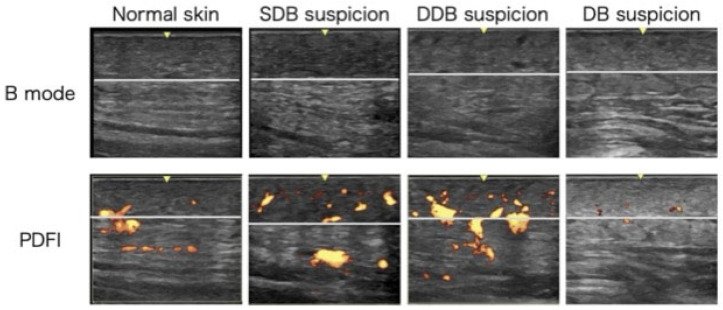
Representative high-frequency ultrasound images of burn wounds. Reproduced under terms of the CC-BY license [105].
